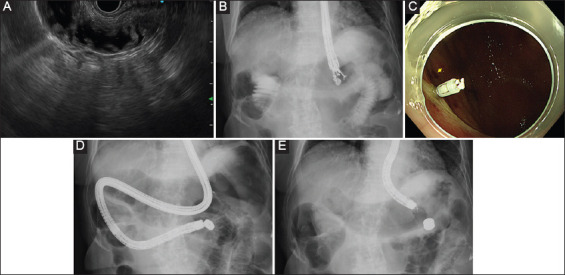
EUS with color Doppler from the stomach revealed the absence of non-target tissue, including small intestine, colon, and large vessels, adjacent to the anastomosis where the magnets were to be placed (A). EUS-guided marking using biopsy forceps by biting the mucosa (arrows) and placing a hemoclip was performed at the anastomosis site in the stomach (B, C). A flat plate-shaped magnet was pushed and delivered to the duodeno-jejuno junction adjacent to the marking hemoclip as an indicator by a forward-viewing endoscope (D). Another flat plate-shaped magnet was delivered endoscopically to the marking point in the stomach using biopsy forceps. The magnets were attracted toward each other transmurally (E).
